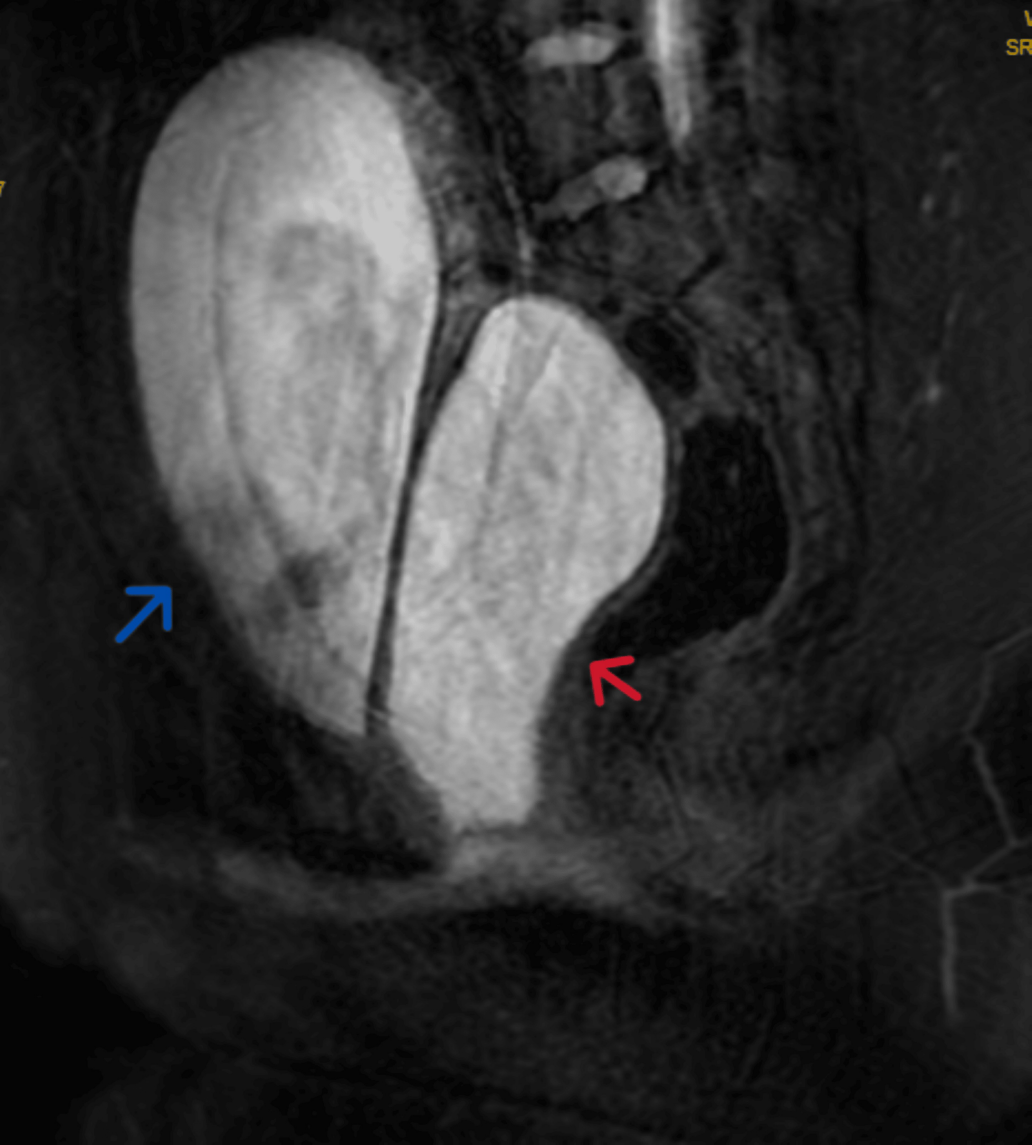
MRI pelvis after partial voiding of urine The blue arrow indicates reduction of the urine volume in the urinary bladder and the red arrow indicates overdistended vagina with urine
